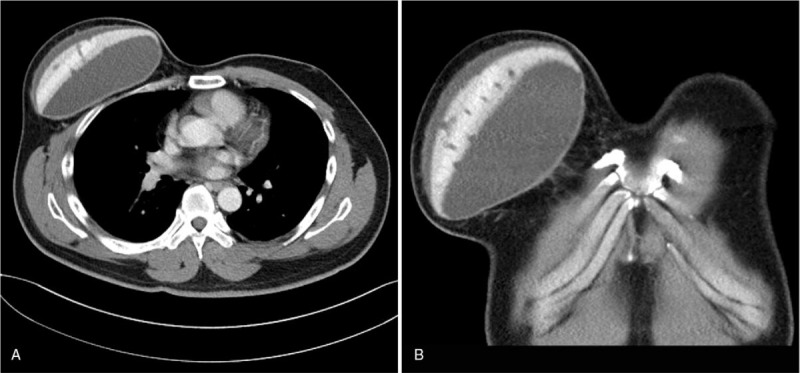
Radiologic finding of the patient. Left, Pectoral muscle is not found on the right side. About 4 mm-thickness rim enhancement under the subcutaneous tissue is noted, suggesting prominent capsule formation. Internal medium signal intensity suggests a large amount of fluid collection. Inside the capsule, the custom-made implant is noted. Right, Coronal view of the CT, showing the largest diameter of the implant measureing over 16 cm. Evenly spaced punched-out lesions are found, which was presumed to be the result of customization.
